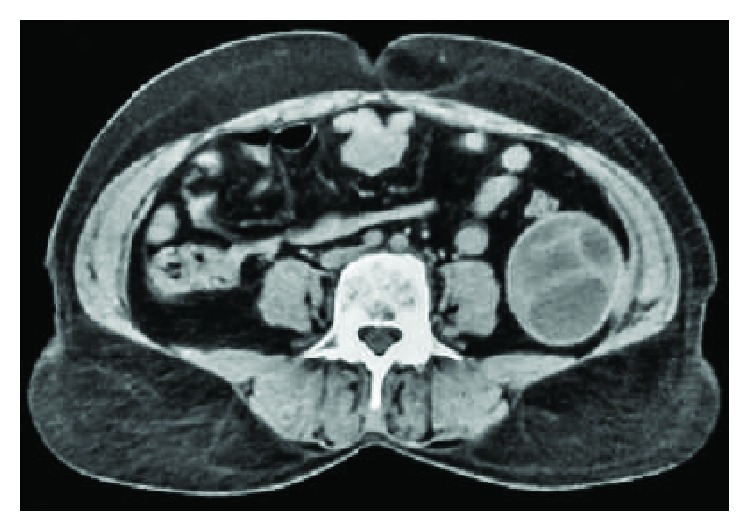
CT scan of the abdomen showing giant multiloculated hydatid cyst with septations in the left iliac fossa.
